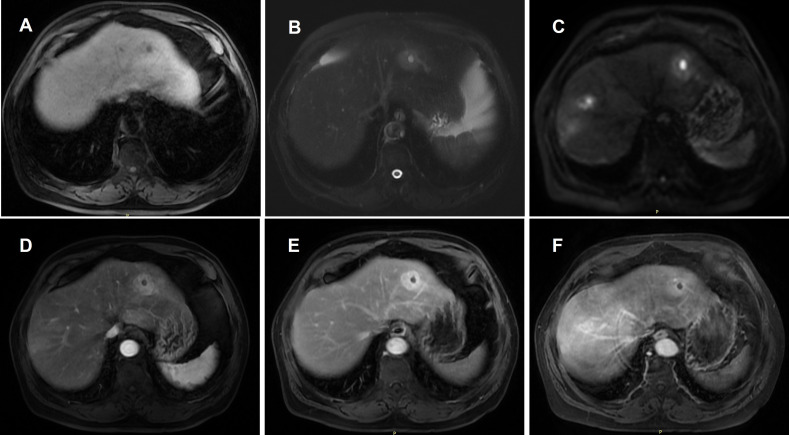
Representative MRI features of multifocal hepatic nodules (left lobe).The lesions exhibit slightly low signal intensity on T1WI (A) and slightly high signal intensity on T2WI (B). Central quasi-circular necrotic areas show low T1 and high T2 signal intensity. DWI (C) demonstrates diffusion restriction predominantly in the solid components of the lesions. Contrast-enhanced images show mild arterial enhancement (D) followed by progressive enhancement in the portal phase (E). The delayed phase (F) demonstrates persistent enhancement that remains slightly hyperintense relative to the surrounding liver parenchyma. No enhancement is observed within the necrotic areas across all phases.
